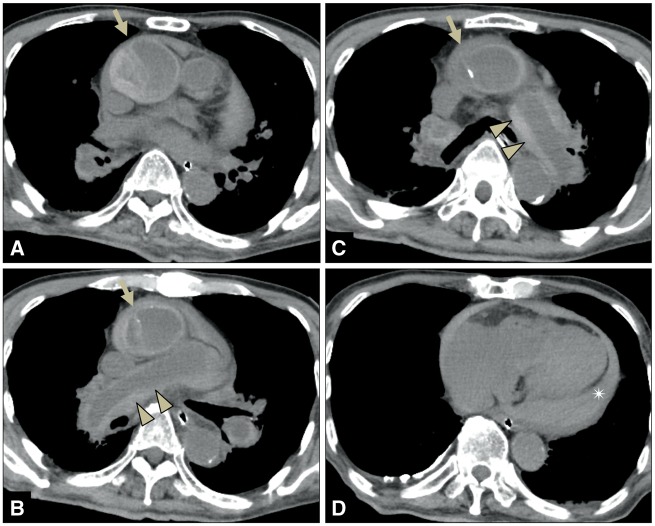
Computed tomography image of Patient 1 after the discontinuation of cardiopulmonary resuscitation shows an intimomedial flap localized to the ascending aorta (*A*-arrow), inward shift of the calcified intima (*B*/*C*-arrows), increased bilateral pulmonary artery wall attenuation (*B*/*C*-arrowheads), and large amount of bloody pericardial effusion (*D*-asterisk). The computed tomography values of bloody pericardial effusion were 40–52 HUs.
